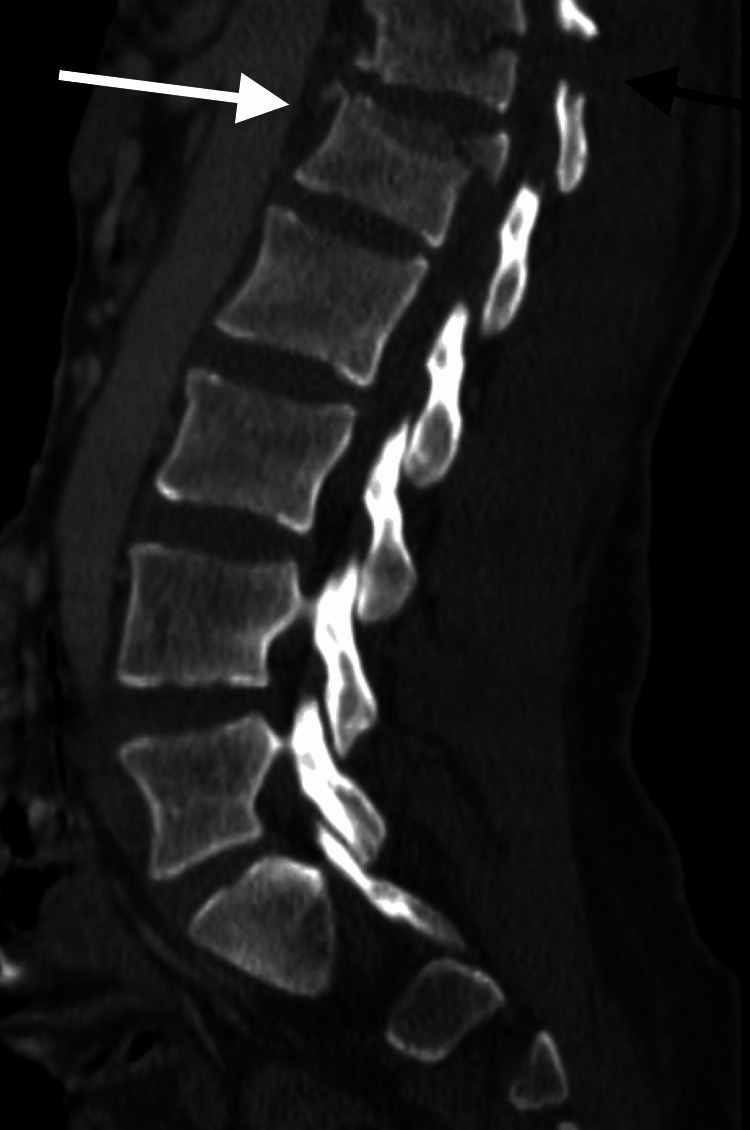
Preoperative sagittal CT for patient 1 demonstrates a bony Chance fracture (AOSpine subtype B1) through T12 (black arrow) and an incomplete burst fracture (AOSpine subtype A3) through the superior portion of L1 (white arrow).
